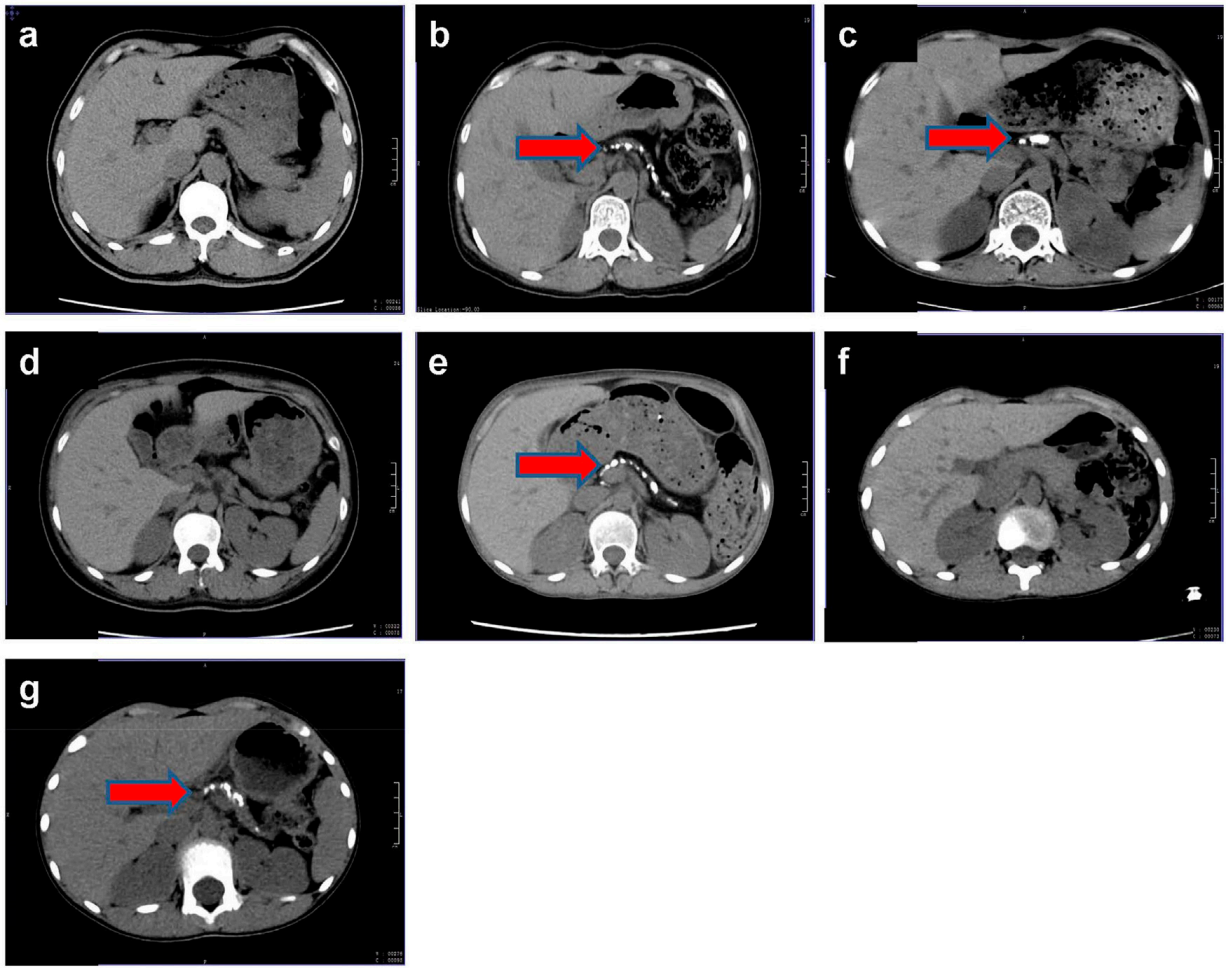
Pancreas CT of this family. (a) The proband’s father, Ia; (b) the proband’s mother, Ib; (c) the proband, IIa; (d) the proband’s first sister, IIb; (e) the proband’s second sister, IIc; (f) the proband’s brother, IId; (g) the proband’s third sister, IIe; red arrow, pancreatic calcification.
